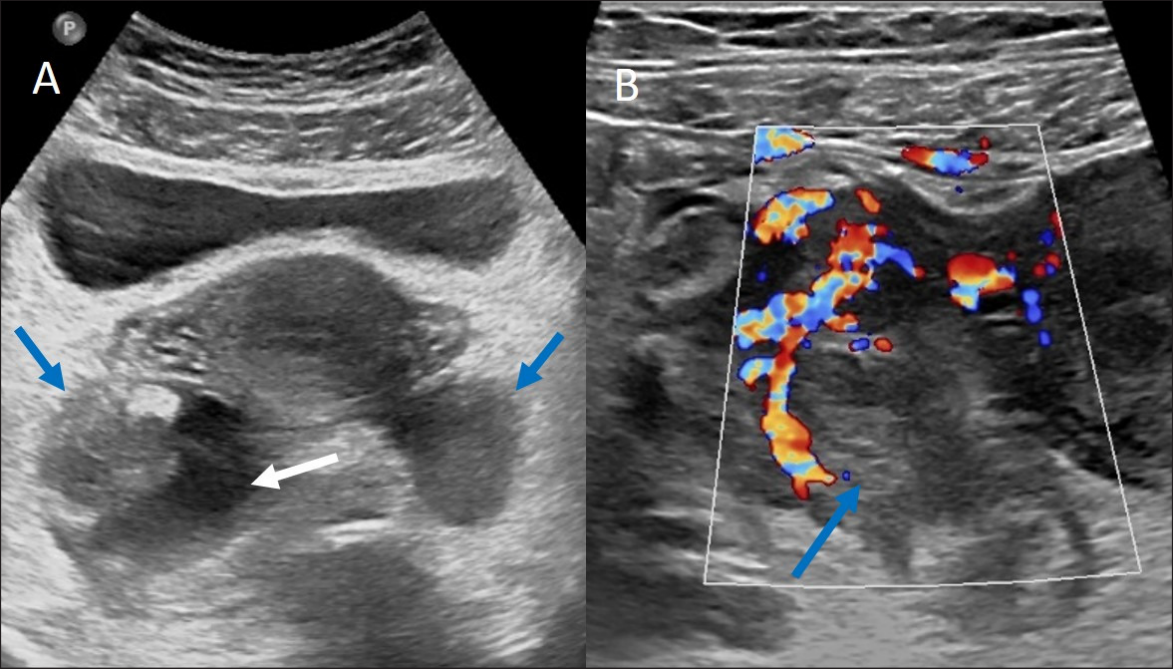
Magnetic resonance imaging (MRI) confirmed the diagnosis of tubo-ovarian abscess. Axial and sagittal T2 weighted images (Figure 2) showed bilateral complex cystic adnexal mass (Figure 2A, blue arrows) with peri-ovarian fat stranding and right fluid-fluid level corresponding to intra-ovarian declive pus (Figure 2A, white arrow). It also revealed dilated fallopian tube filled with complex internal fluid (Figure 2B, blue arrow) corresponding to pyosalpinx.
